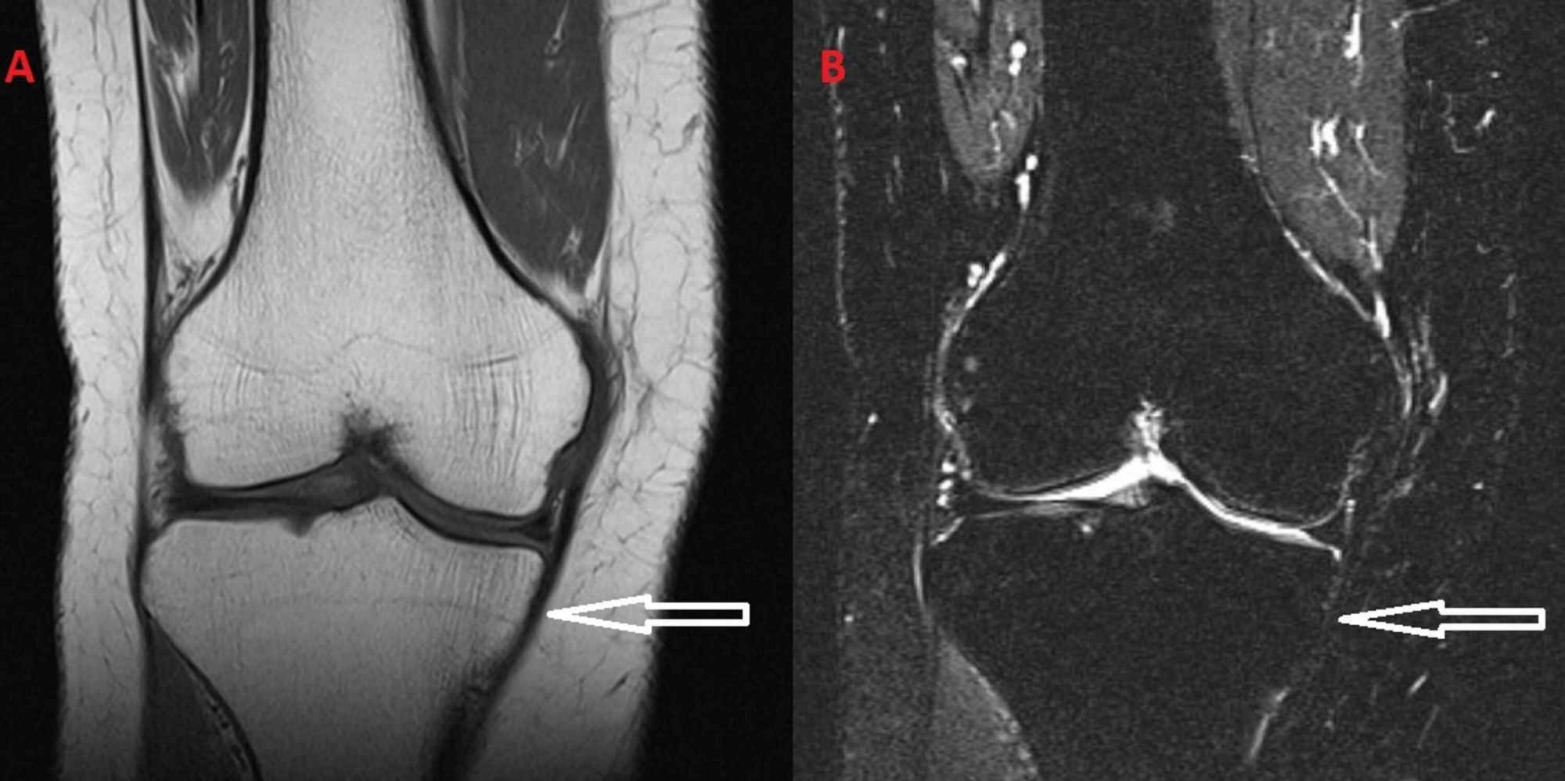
(A) T1-weighted images and (B) T2-weighted images from the MRI after the subsidence of the symptoms.
